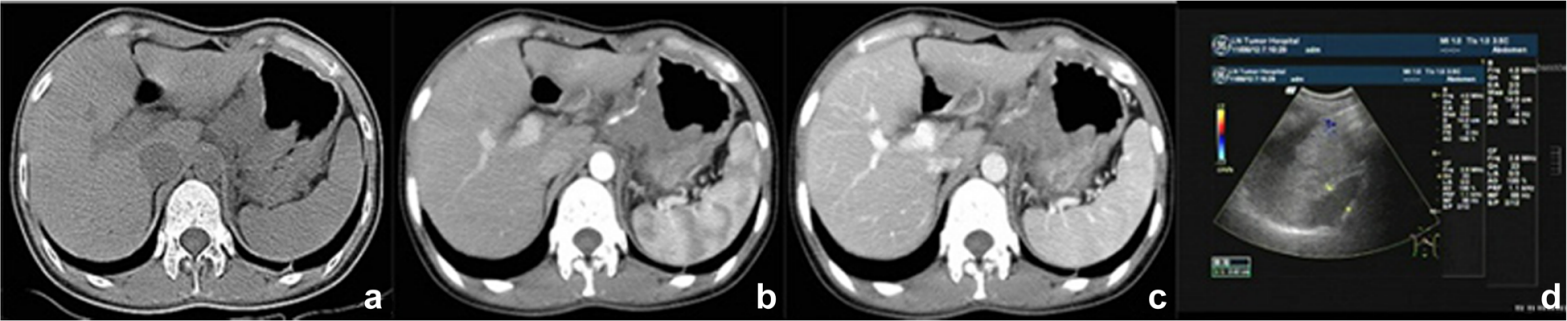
T4 gastric cancer in cardia and gastric fundus. (a-c) CT scanning. The gap around fat tissues disappeared, and the lesion invaded other structures such as the tail of the pancreas, left gastric artery. (d) Ultrasound imaging. It was visible that lesions invaded surrounding organs.
